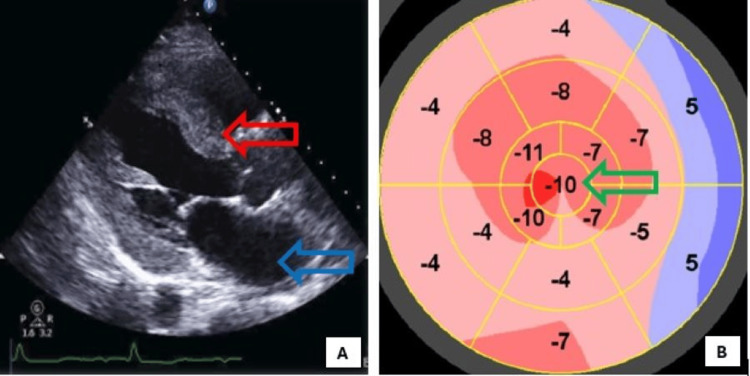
Transthoracic echocardiogram showing left atrial enlargement (blue arrow), left ventricular hypertrophy (red arrow) (A), with diffuse hypokinesia, severe left ventricular systolic dysfunction with an ejection fraction of 10%-15% (on cine images), and reduced global longitudinal strain with some apical sparing (green arrow) (B) in end-stage cardiac amyloidosis.
